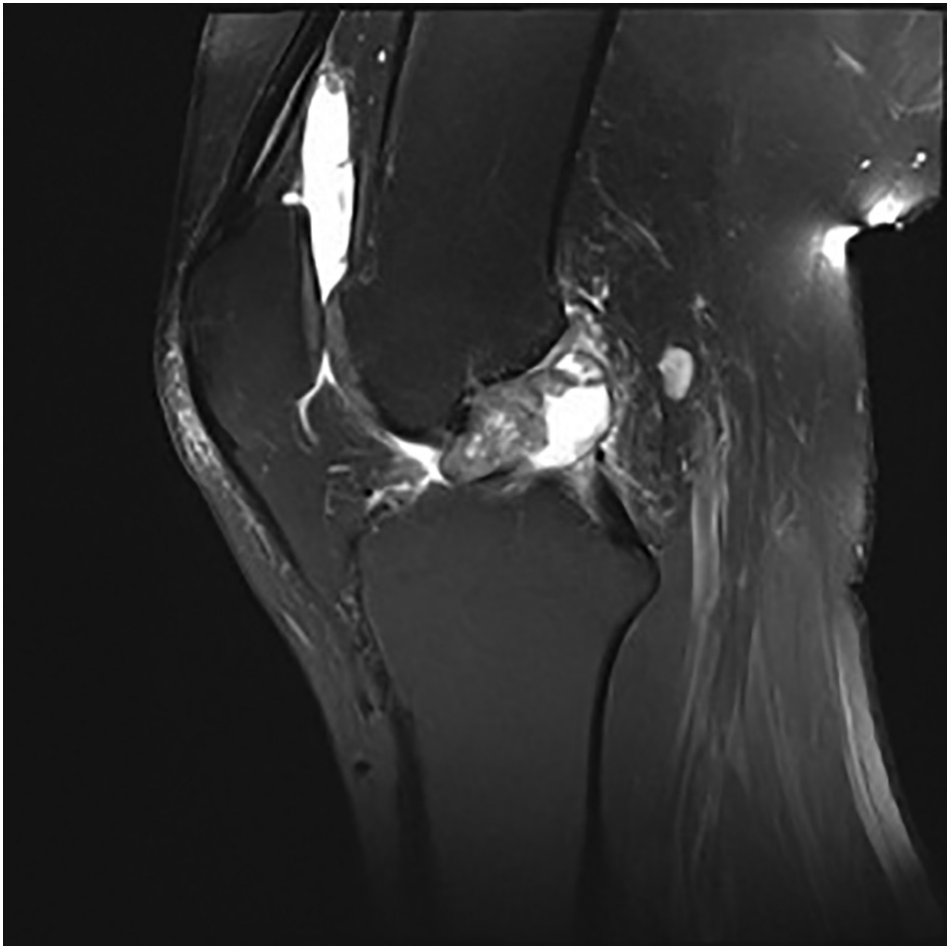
Sagittal T3 MRI image (proton density fat saturation) of knee showing large mass filling the notch of the knee and covering the ACL.
